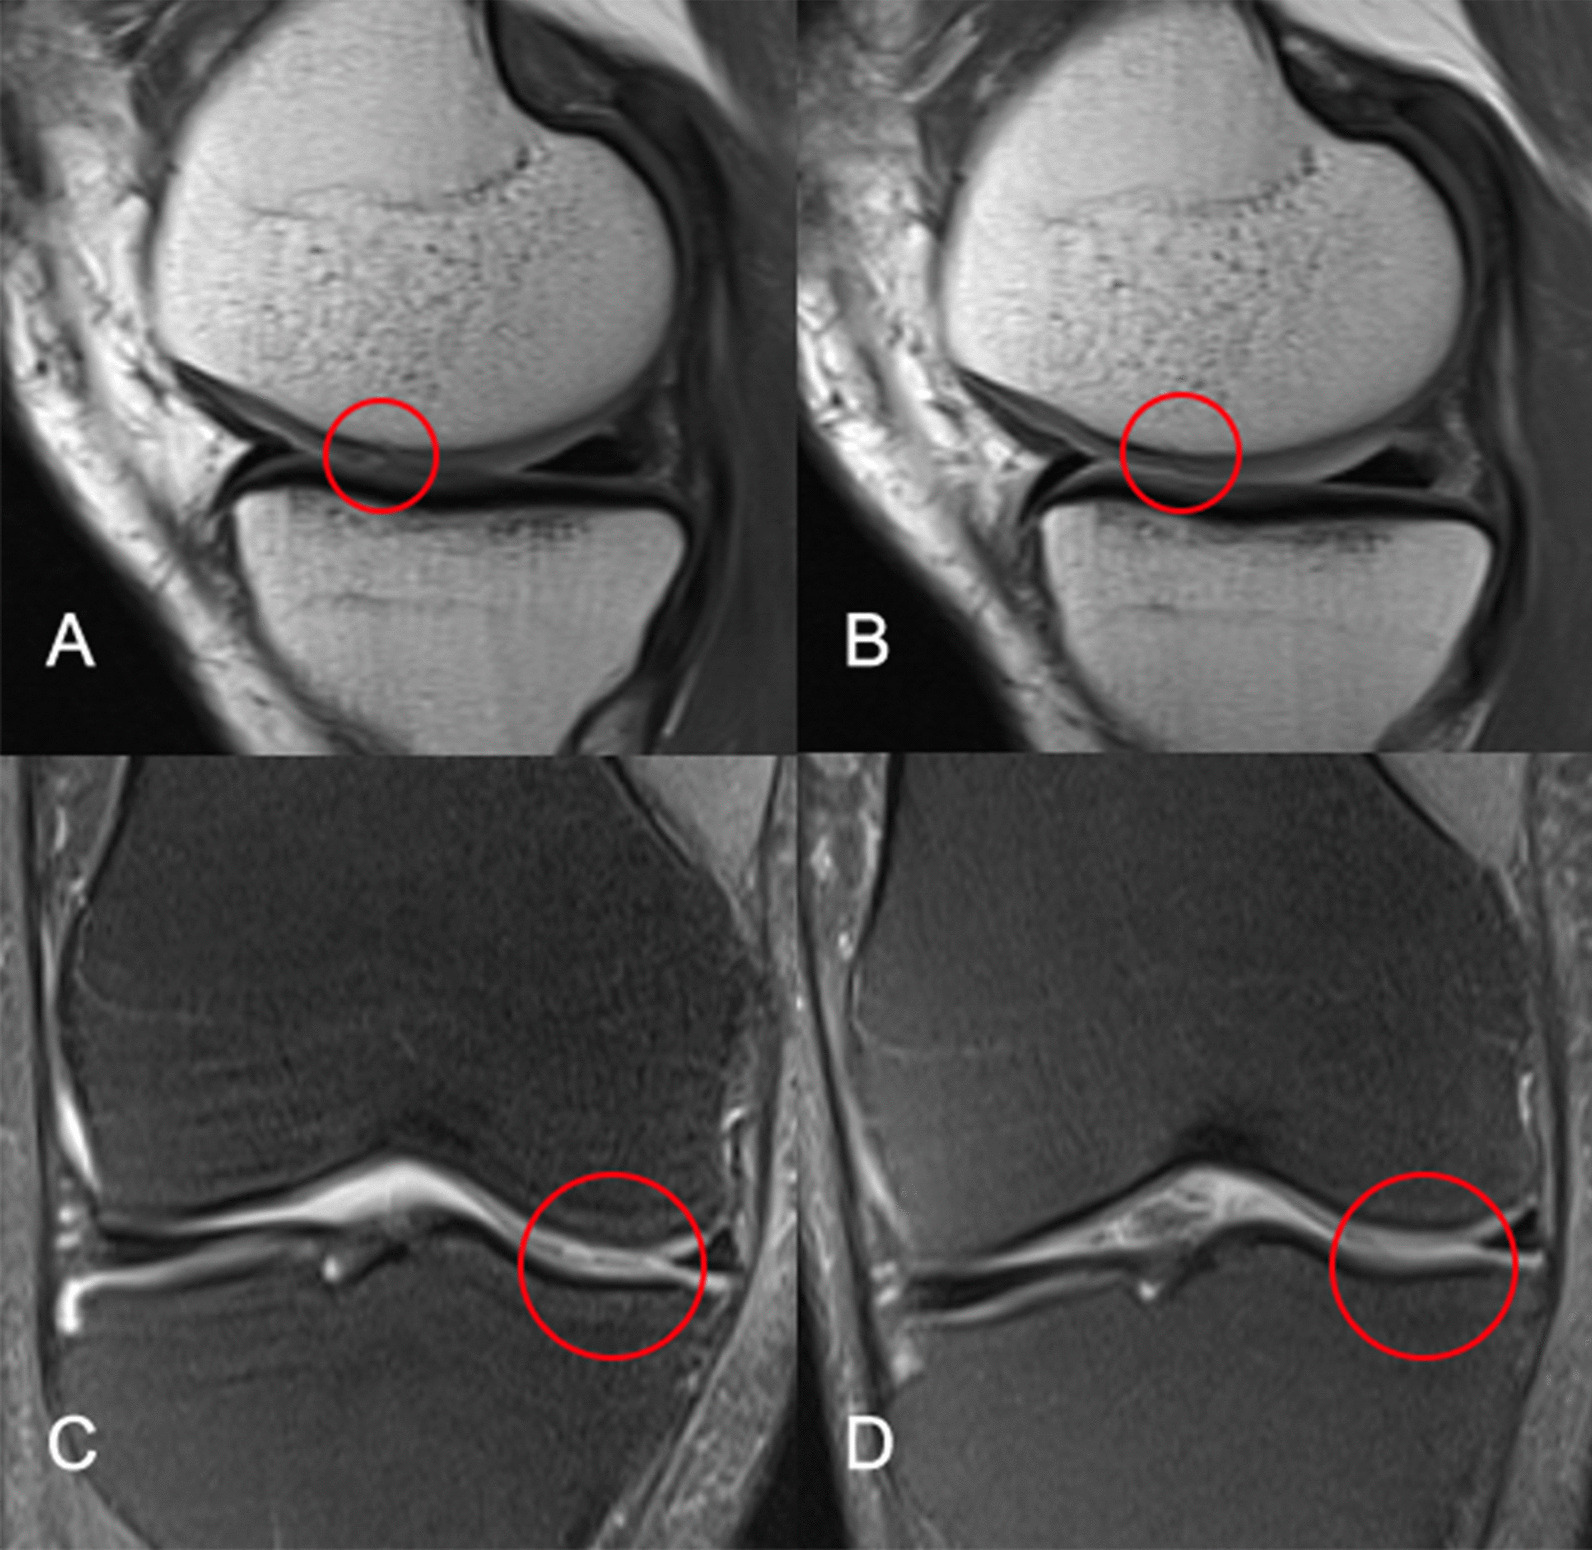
MRI evaluation of full-thickness cartilage defect changes at 5 years. A, C Coronal and sagittal images of the medial femur and tibia before injection of SVF. A grade 1 full-thickness cartilage defect can be observed in the circle. B Coronal and sagittal images of the medial femur and tibia 5 years after SVF injection. The full-thickness cartilage defect in the circled area disappeared, and the cartilage edge was smooth
